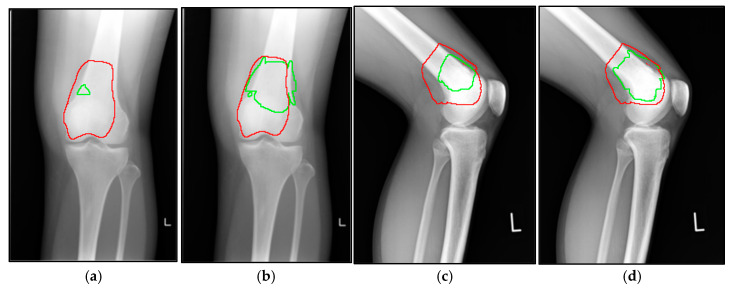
Comparison of lesion detection performance between 1C and 3C AI models in identifying osteosarcoma on X-ray images: (a,b) anteroposterior radiographs showing osteosarcoma detection results of (a) 1C model and (b) 3C model outcomes, where the red line represents the osteosarcoma area (as determined by expert human annotation), and the green line indicates the lesion area identified by the AI model; (c,d) lateral radiographs showing the same comparison, namely (c) 1C model and (d) 3C model outcomes, with red lines representing expert human annotation and green lines showing AI model detection. “L” indicates the left side of the patient.
